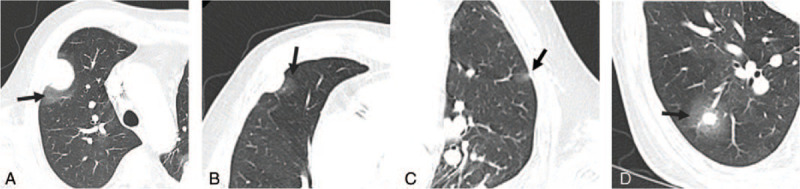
Computed tomography (CT) of the chest at admission. (A–D) The lung setting images showed multiple pleural metastases in the bilateral hemithorax and pulmonary metastasis in right lower lobe. The lung setting images demonstrated that each nodule was surrounded by a ground glass opacity with a halo sign (black arrow) representing hypervascular metastases.
